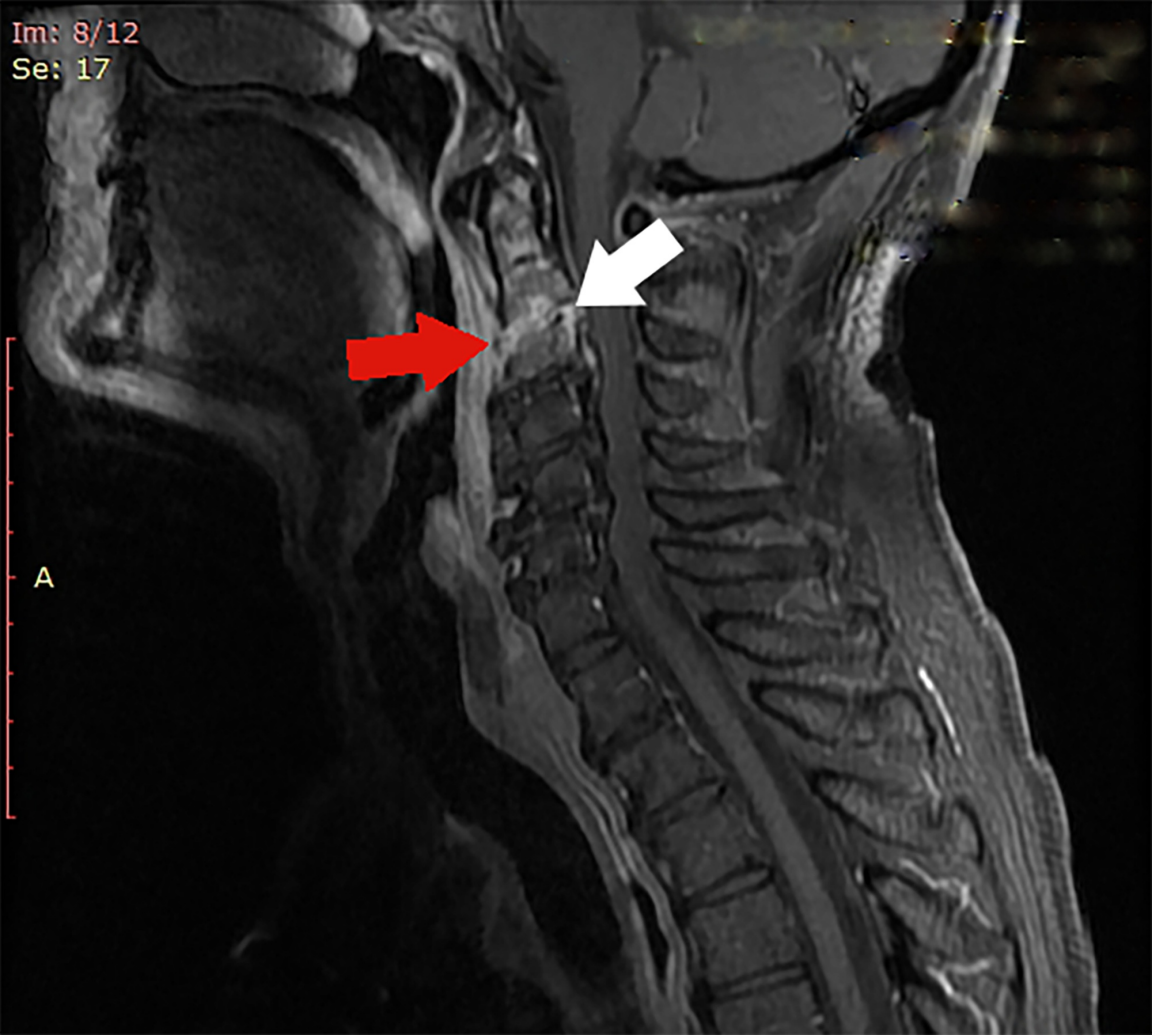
MRI scan MRI that shows C2/3 spondylodiscitis (red arrow) and epidural abscess with cord compression (white arrow)
